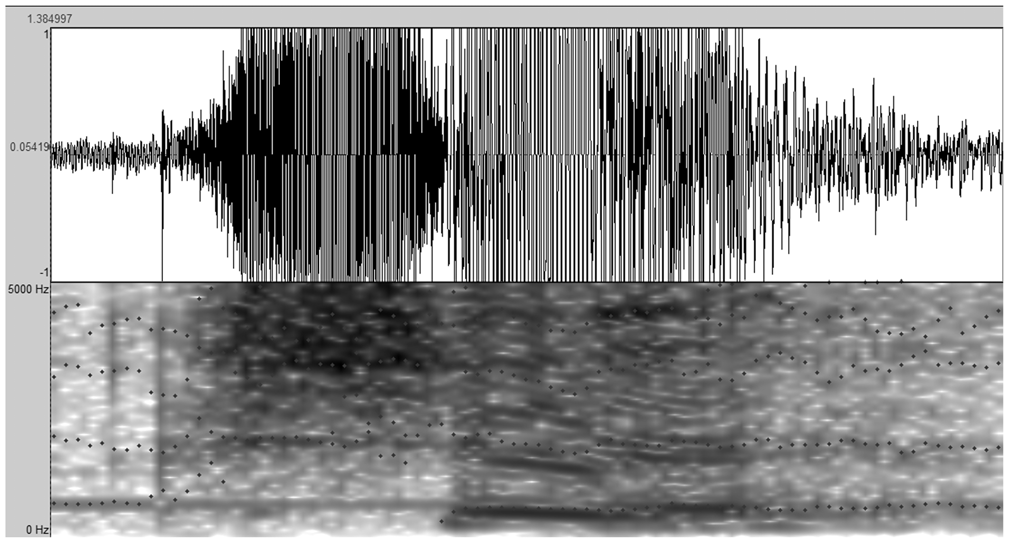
Sample sonogram as provided by Praat 5.1.30 software.
